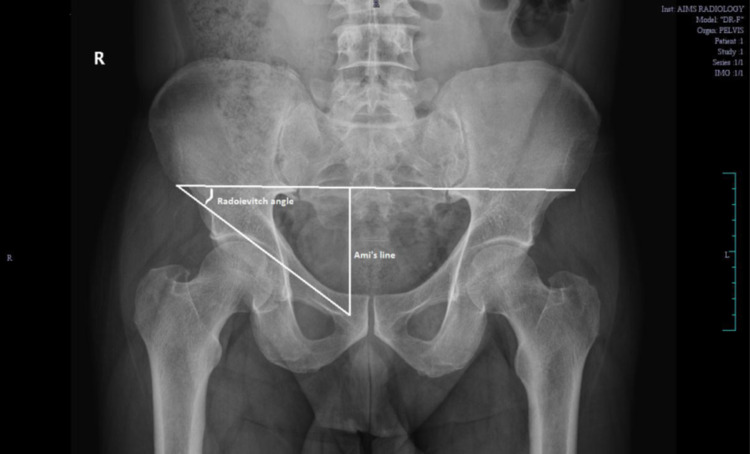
Plain Radiograph of the Pelvis with Bilateral Hip Depicting Radoievitch's angle and Ami's Line
